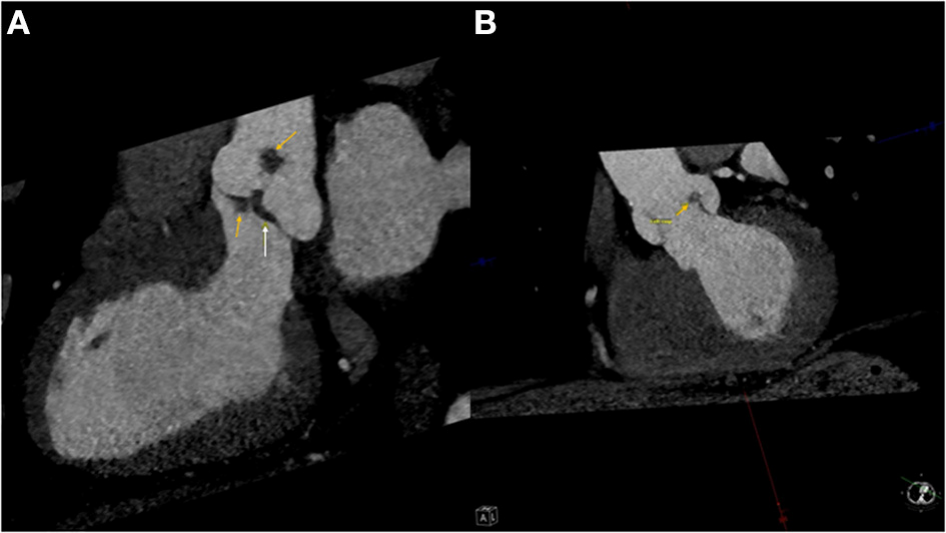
(A) Left ventricular outflow tract view of the pre-operative CT angiogram showing the masses (Yellow arrows) and the thickening of the non-coronary cusp of the aortic valve (White arrow). (B) An XX view of the aortic valve with indicating the mass on the left cusp of the aortic valve (Yellow arrow).
